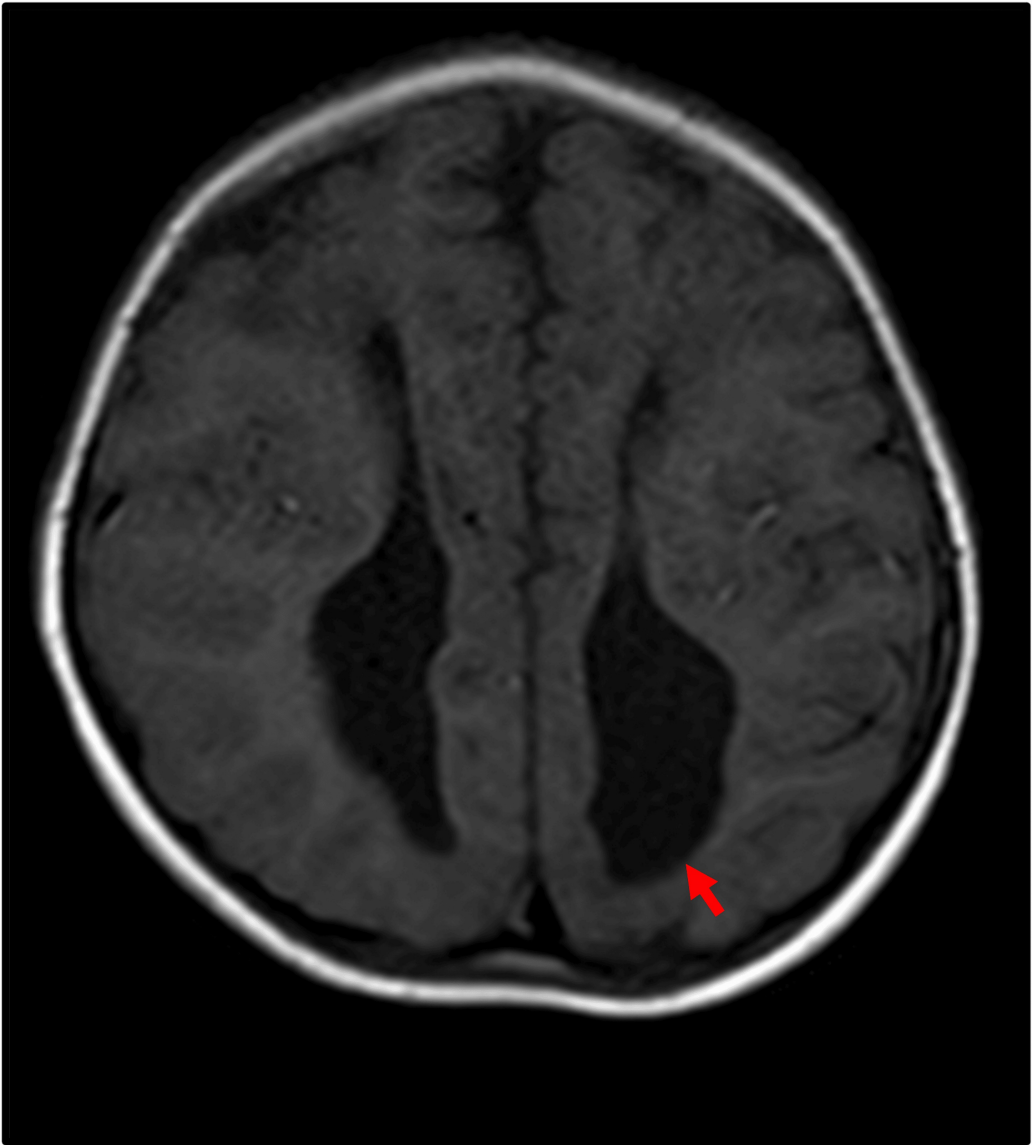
Agenesis of the corpus callosum with colpocephaly. Axial T1-weighted imaging showing the parallel orientation of the lateral ventricles with dilated trigones and occipital horns (red arrow).
